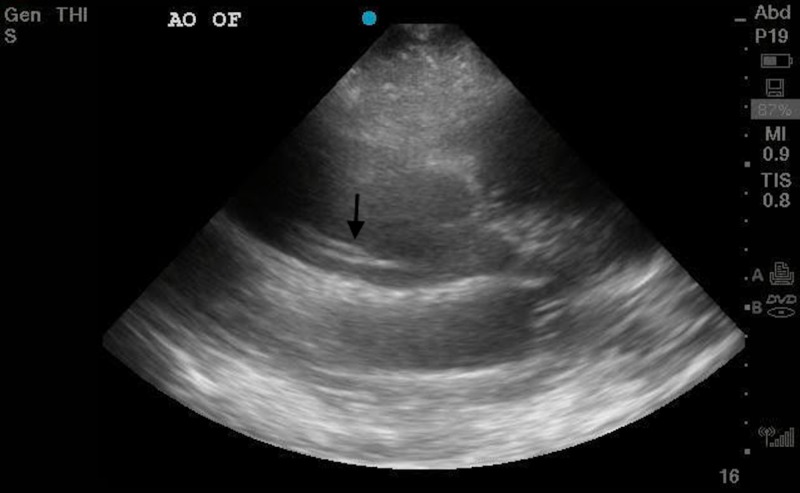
Ultrasound image of an intimal flap by the aortic root
